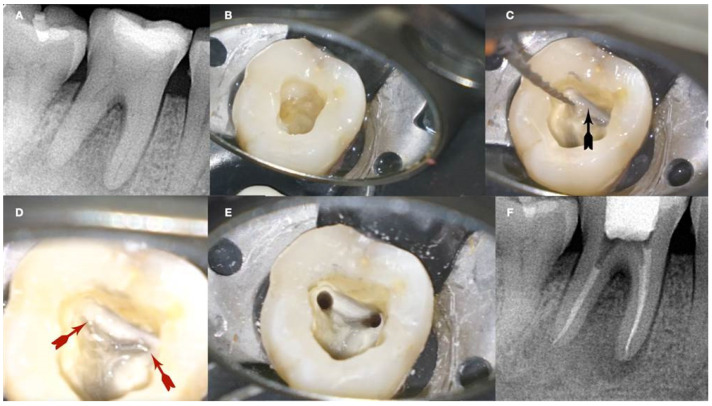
Calcified mandibular molar negotiation (A). Preoperative radiograph of a calcified mandibular molar with no visible canals (B). Clinical view of the calcified chamber (C). Clinical view of the calcified tissue blocking the access to the mesial canal system. Notice the white color of the calcification. The axial walls are yellow, and the pulp floor is always grey (D). Clinical view of the pulp floor developmental lines after the removal of the white calcified tissue. Chasing the dark grey developmental lines to their termini will reveal the calcified canal entrances (E). Clinical view of the canals shaped (F). Postoperative radiograph of the calcified mandibular molar canal treatment (clinical images and radiographs courtesy of Dr. Chaniotis Antonis). Black arrow: calcified structure covering the canal orifices and the developmental pulp chamber lines, red arrows indicate the canal orifices after the removal of the white calcified structure (black arrow).
